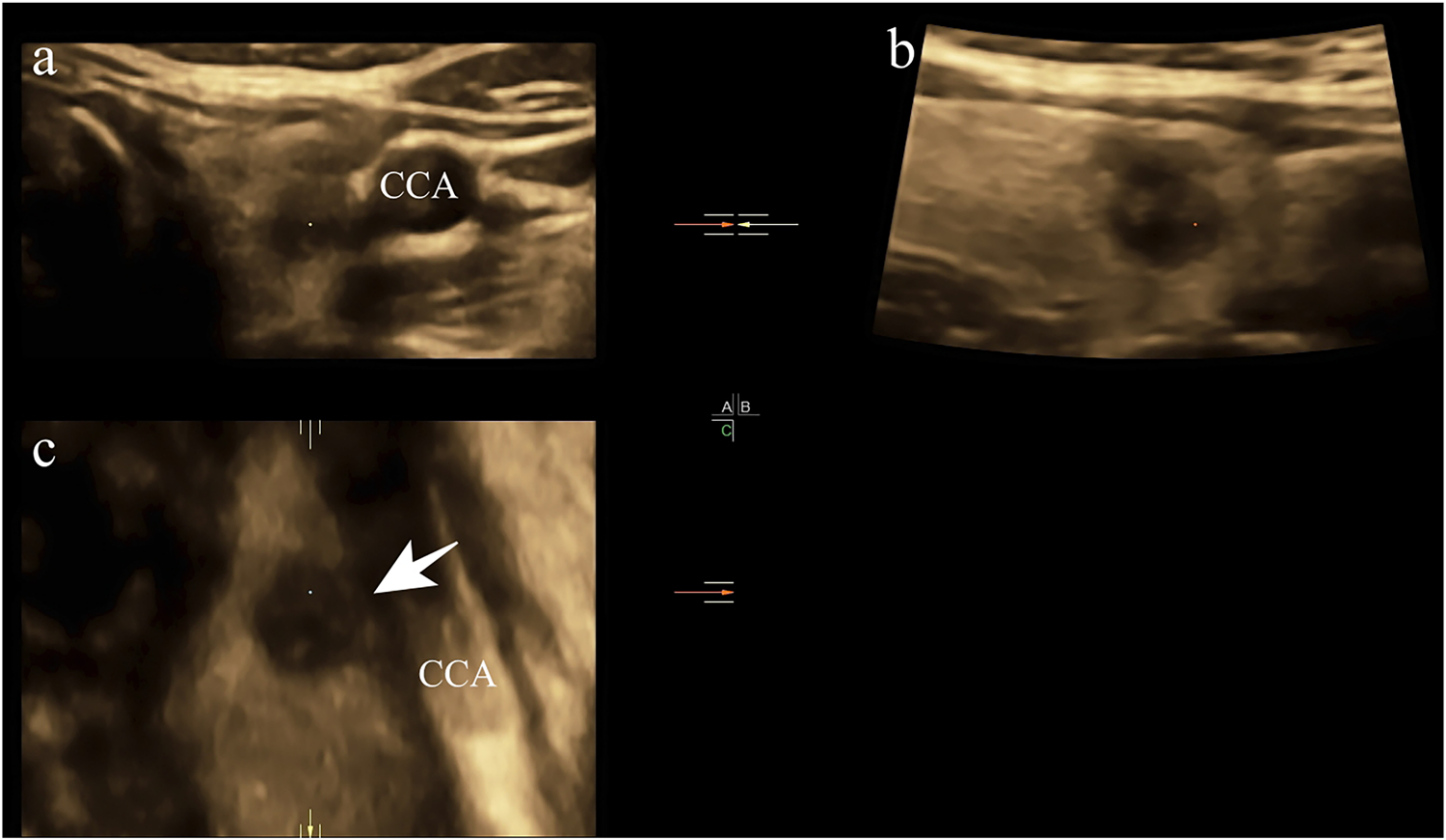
The multiplane of 3D-US showing the transverse (a), longitudinal (b), and coronal planes (c) of the above thyroid nodule in Fig. 1. In the c plane, the thyroid capsule near the CCA was interrupted (white arrow). 3D-US, three-dimensional ultrasound; CCA, common carotid artery
